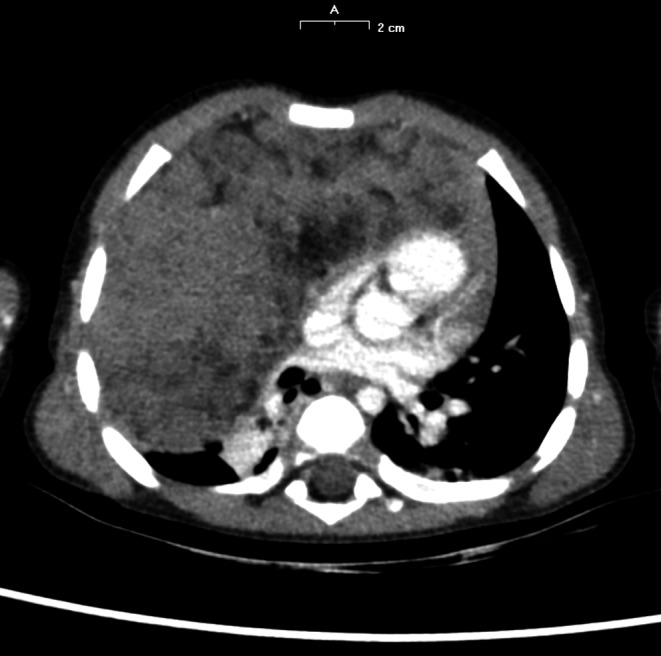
Chest CT scan showing the large mediastinal mass
